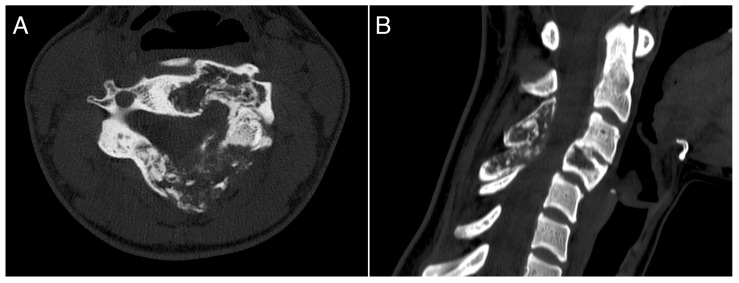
CT scan performed at last follow-up 24 months after denosumab re-challenge. (A): axial view; (B): sagittal view.
